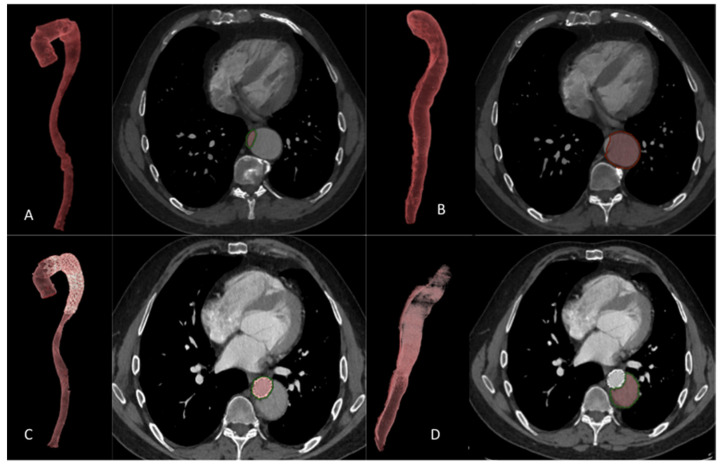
Aortic volume measurement. Aortic volume measurement after true lumen (A–C) selection and false lumen (B–D) selection: (A,B): preoperative CT scan (C,D): postoperative CT scan.
